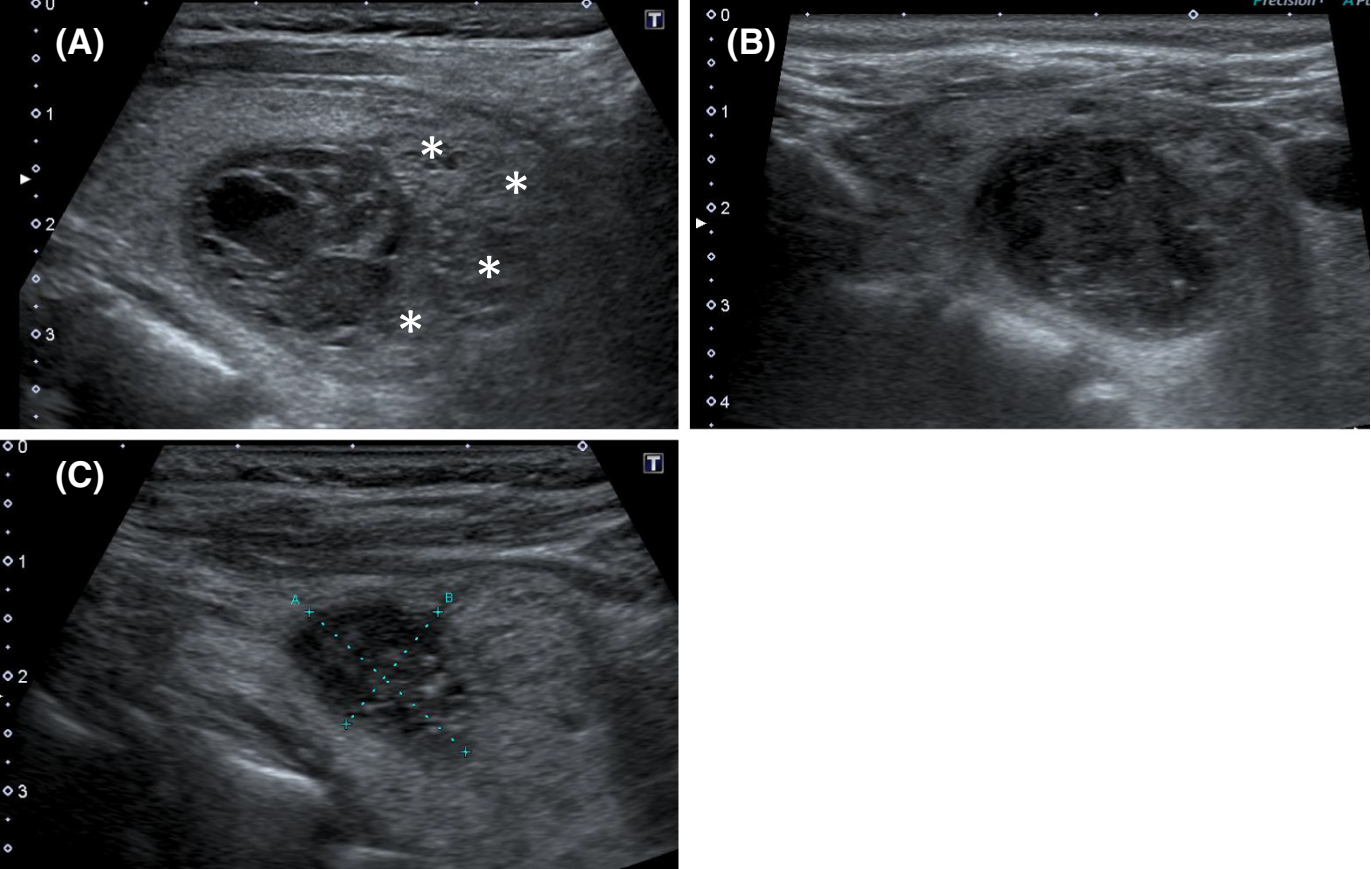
Ultrasonography of the left lobe of the thyroid gland. (A) On admission, multiple preexisting multigoiter nodules were observed (asterisks). Notably, a round, hypoechoic lesion with heterogeneous internal echogenicity was observed. This lesion lacked a distinct capsule and measured approximately 26 × 22 mm in diameter. (B) Ultrasonography on day 6. The hypoechoic lesion had increased in size (29 × 27 mm), and its internal echogenicity was lower than that at the time of admission. (C) Ultrasonography 1 month after discharge. The size of the hypoechoic lesion had become obviously diminished (17 × 15 mm).
